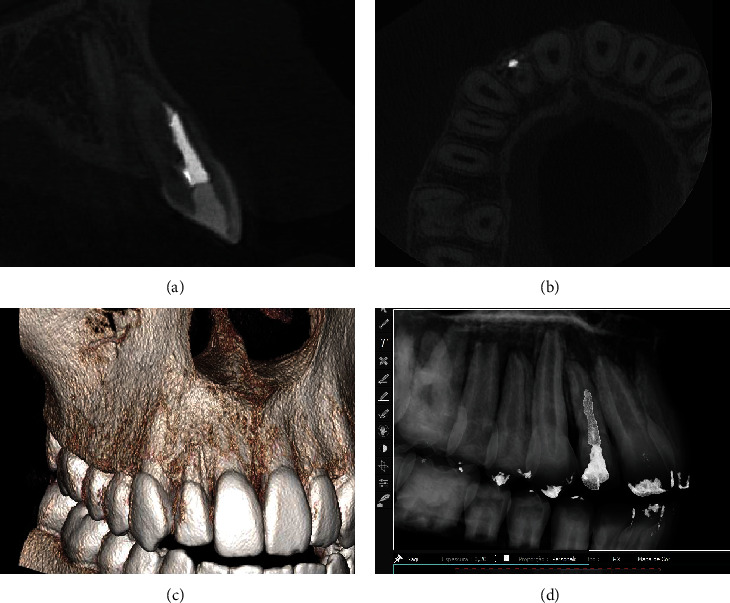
(a–d) CBCT scan and 3D reconstructions two years after the surgery show healing process of the buccal bone and absence of signs of inflammation in the root apex.
